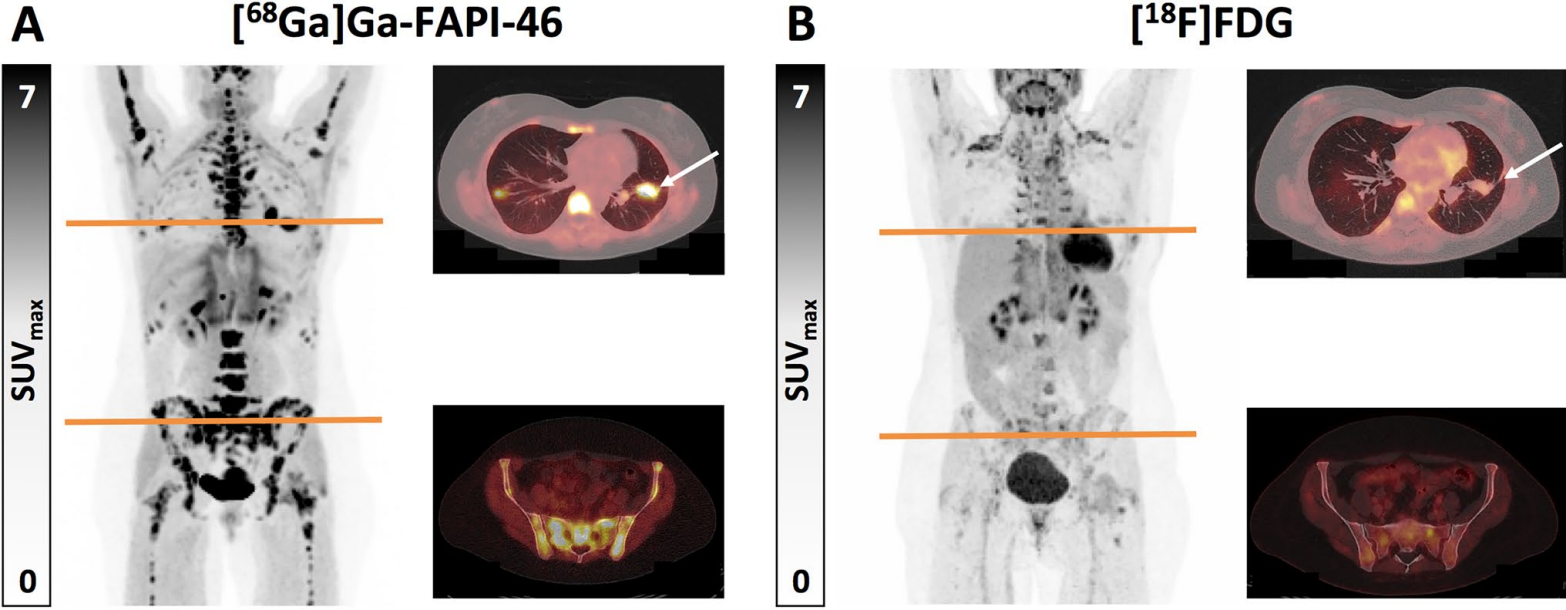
Fifty-two year old female patient who presented a tumour in the right dorsal thigh that had been surgically resected, the pathology study labelling it as a solitary fibrous tumour corresponding to the primary tumour. Re-staging with [^68^Ga]Ga-FAPI-46 PET/CT and [^18^F]FDG PET/CT was performed, detecting pulmonary metastases and diffuse osseous metastases with significantly increased uptake on [^68^Ga]Ga-FAPI-46 PET/CT (A; white arrow, SUVmax 16.7) and low uptake on [^18^F]FDG PET/CT (B; white arrow, SUVmax 3.1)
